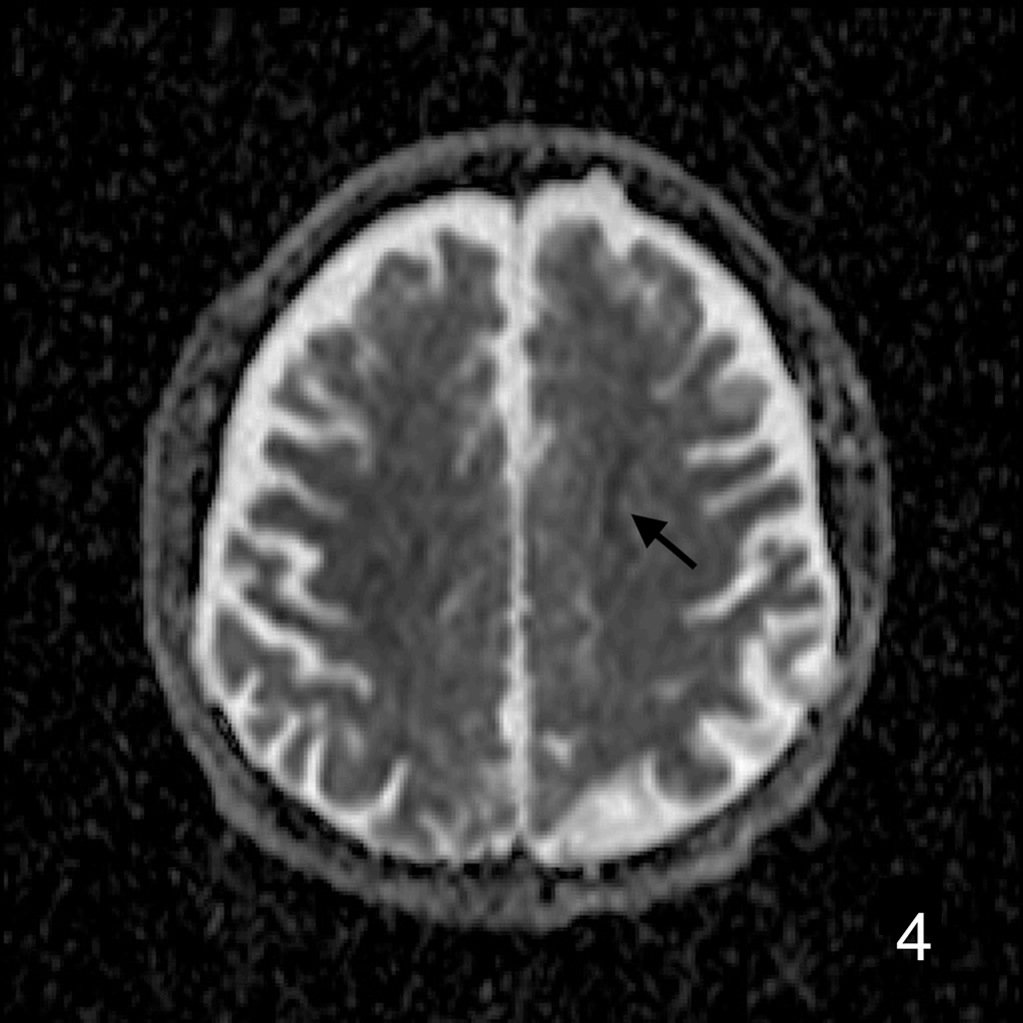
Axial DWI-ADC images on 3^rd^ day shows restriction at cerebral hemisphere.
